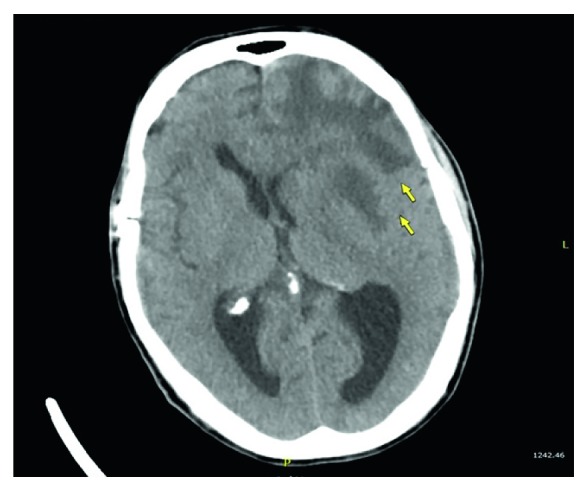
CT brain axial view demonstrating left frontal lobe mass concerning for glioblastoma.
